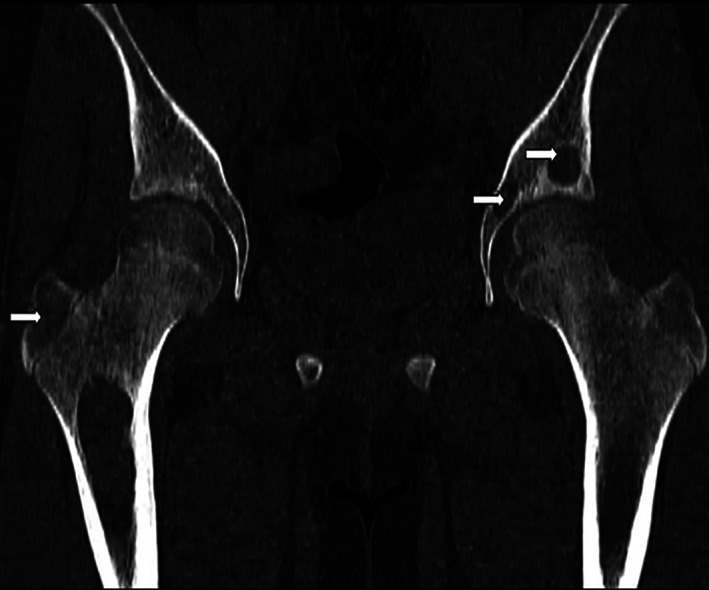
Coronal computed tomography image in bone window shows multiple geographical lytic lesions in bones in right femur and left pelvic bones (white arrows)
